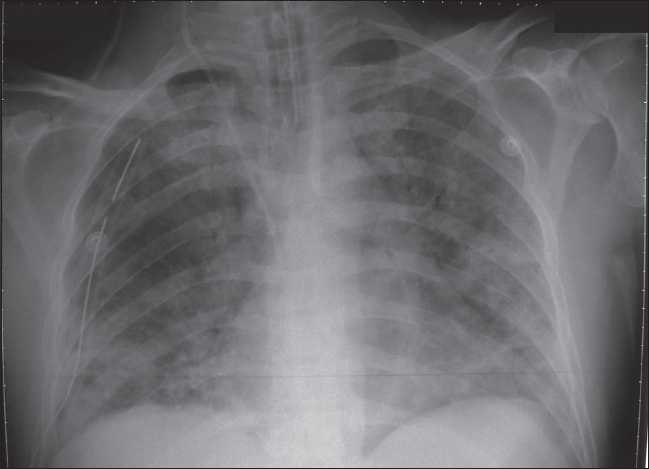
X-ray showed bilateral diffuse infiltrates
